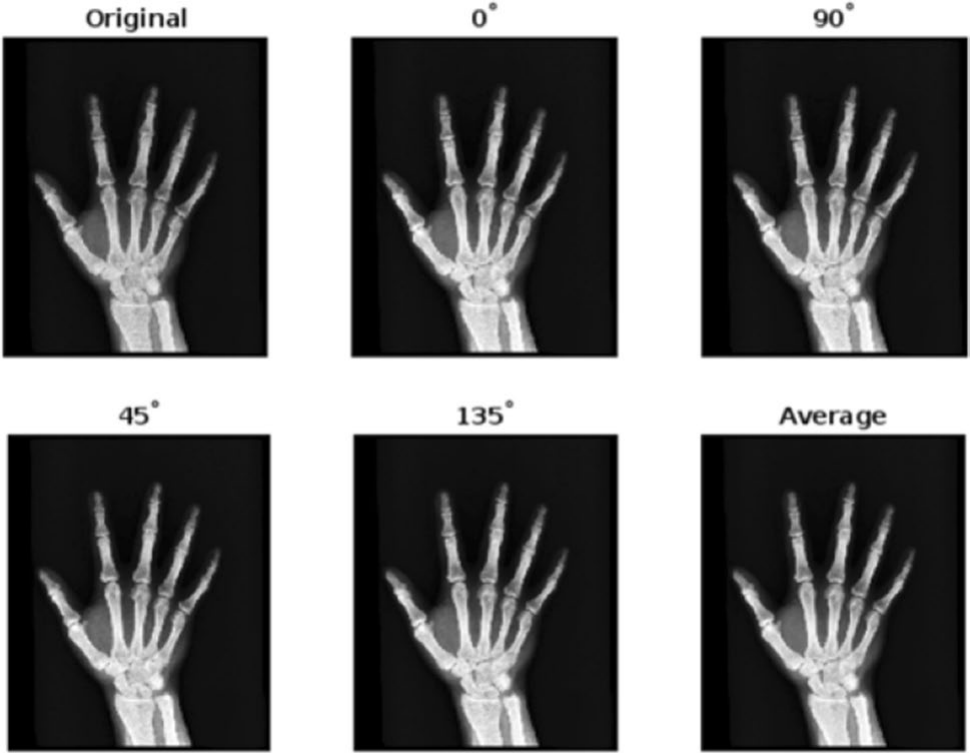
Enhancement of “X-RAY” at various mask angles.
